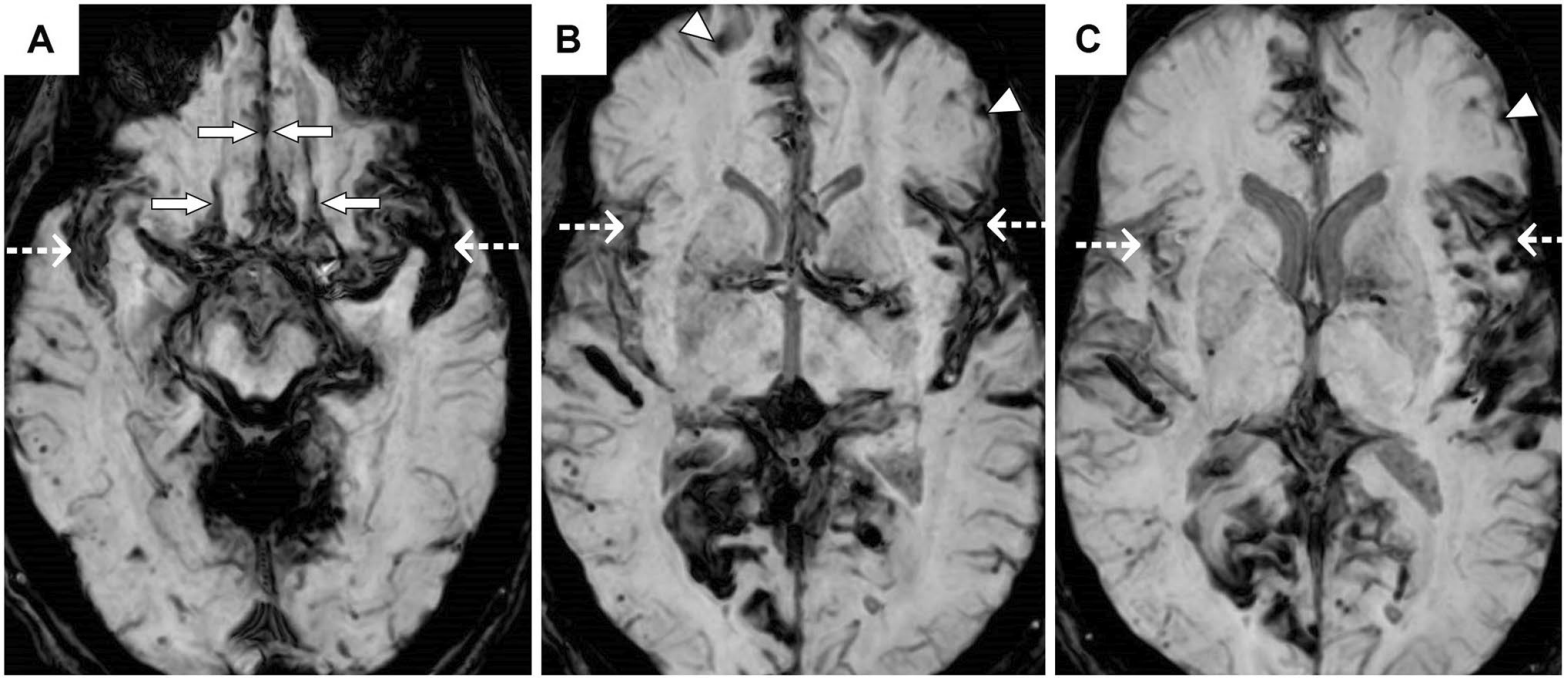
Axial susceptibility-weighted magnetic resonance images of the brain demonstrating haemosiderin deposits lining the orbito-frontal regions (marked with solid arrows, A), Sylvian fissures (marked with dashed arrows, A–C) and the frontal lobe convexities (marked with arrowheads, B, C)
